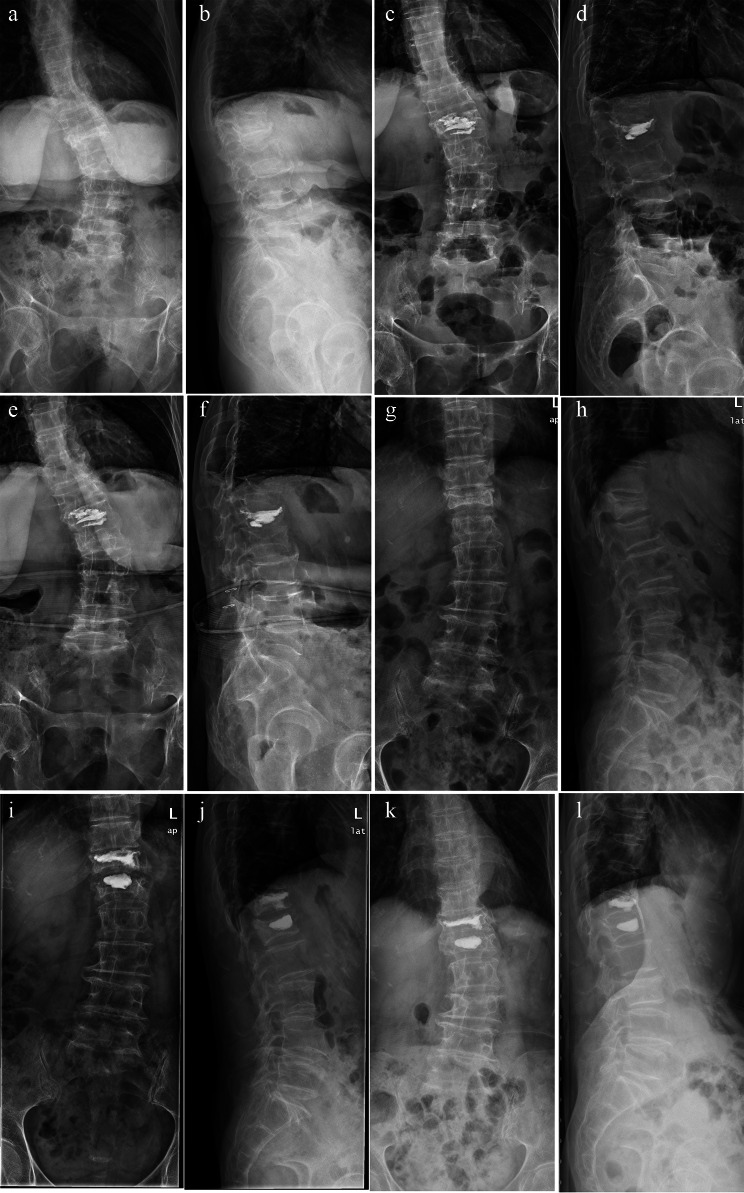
a–f. A 65-year-old female patient with a primary diagnosis of L1 OVCF (2020-12-14) and degenerative scoliosis (T12–L4). a, b. The X-ray film showed L1 vertebral fracture and degenerative scoliosis (Cobb angle of 24°) before surgery. c, d. The X-ray film showed that the Cobb angle was 21° one day after PKP. Although the postoperative angle had decreased slightly, it was still greater than 20°. e, f. The X-ray film showed a refracture of the L2 vertebra and a Cobb angle of 27° for scoliosis after 14 months. g–l. A 69-year-old female patient with a primary diagnosis of T12 and L1 OVCF (2016-8-22) and degenerative scoliosis (T12–L5). 5 g, h. The X-ray film showed that Cobb angle was 16° before surgery. 5 i, j. The X-ray film showed that the Cobb angle was 19° one day after PKP. 5 k, l. The X-ray film showed a refracture of the L3 vertebra and a Cobb angle of 21° for scoliosis after 17 months
